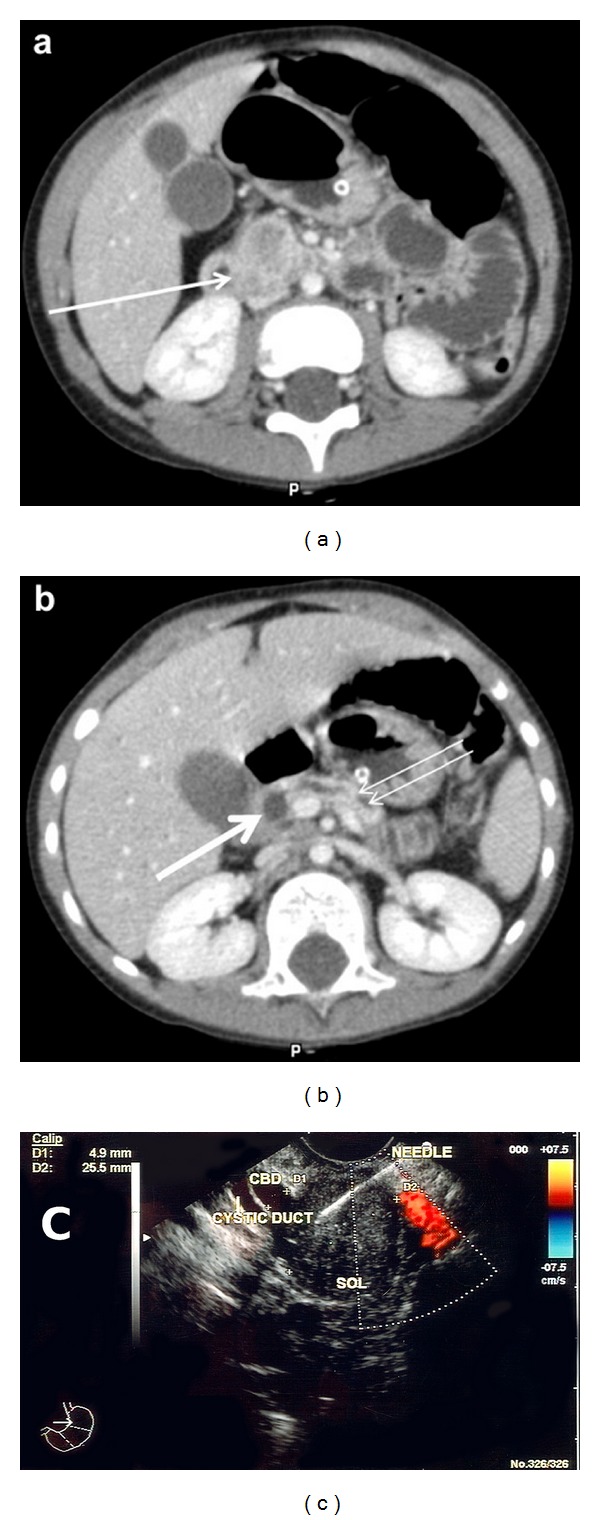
(a) CT Scan of Abdomen: Mass (Arrow) in head of pancreas. (b) CT Scan of Abdomen: Dilated Common (large arrow) and Pancreatic (Double arrow) ducts. (c) Endoscopic ultrasound image of lesion in head of pancreas.
